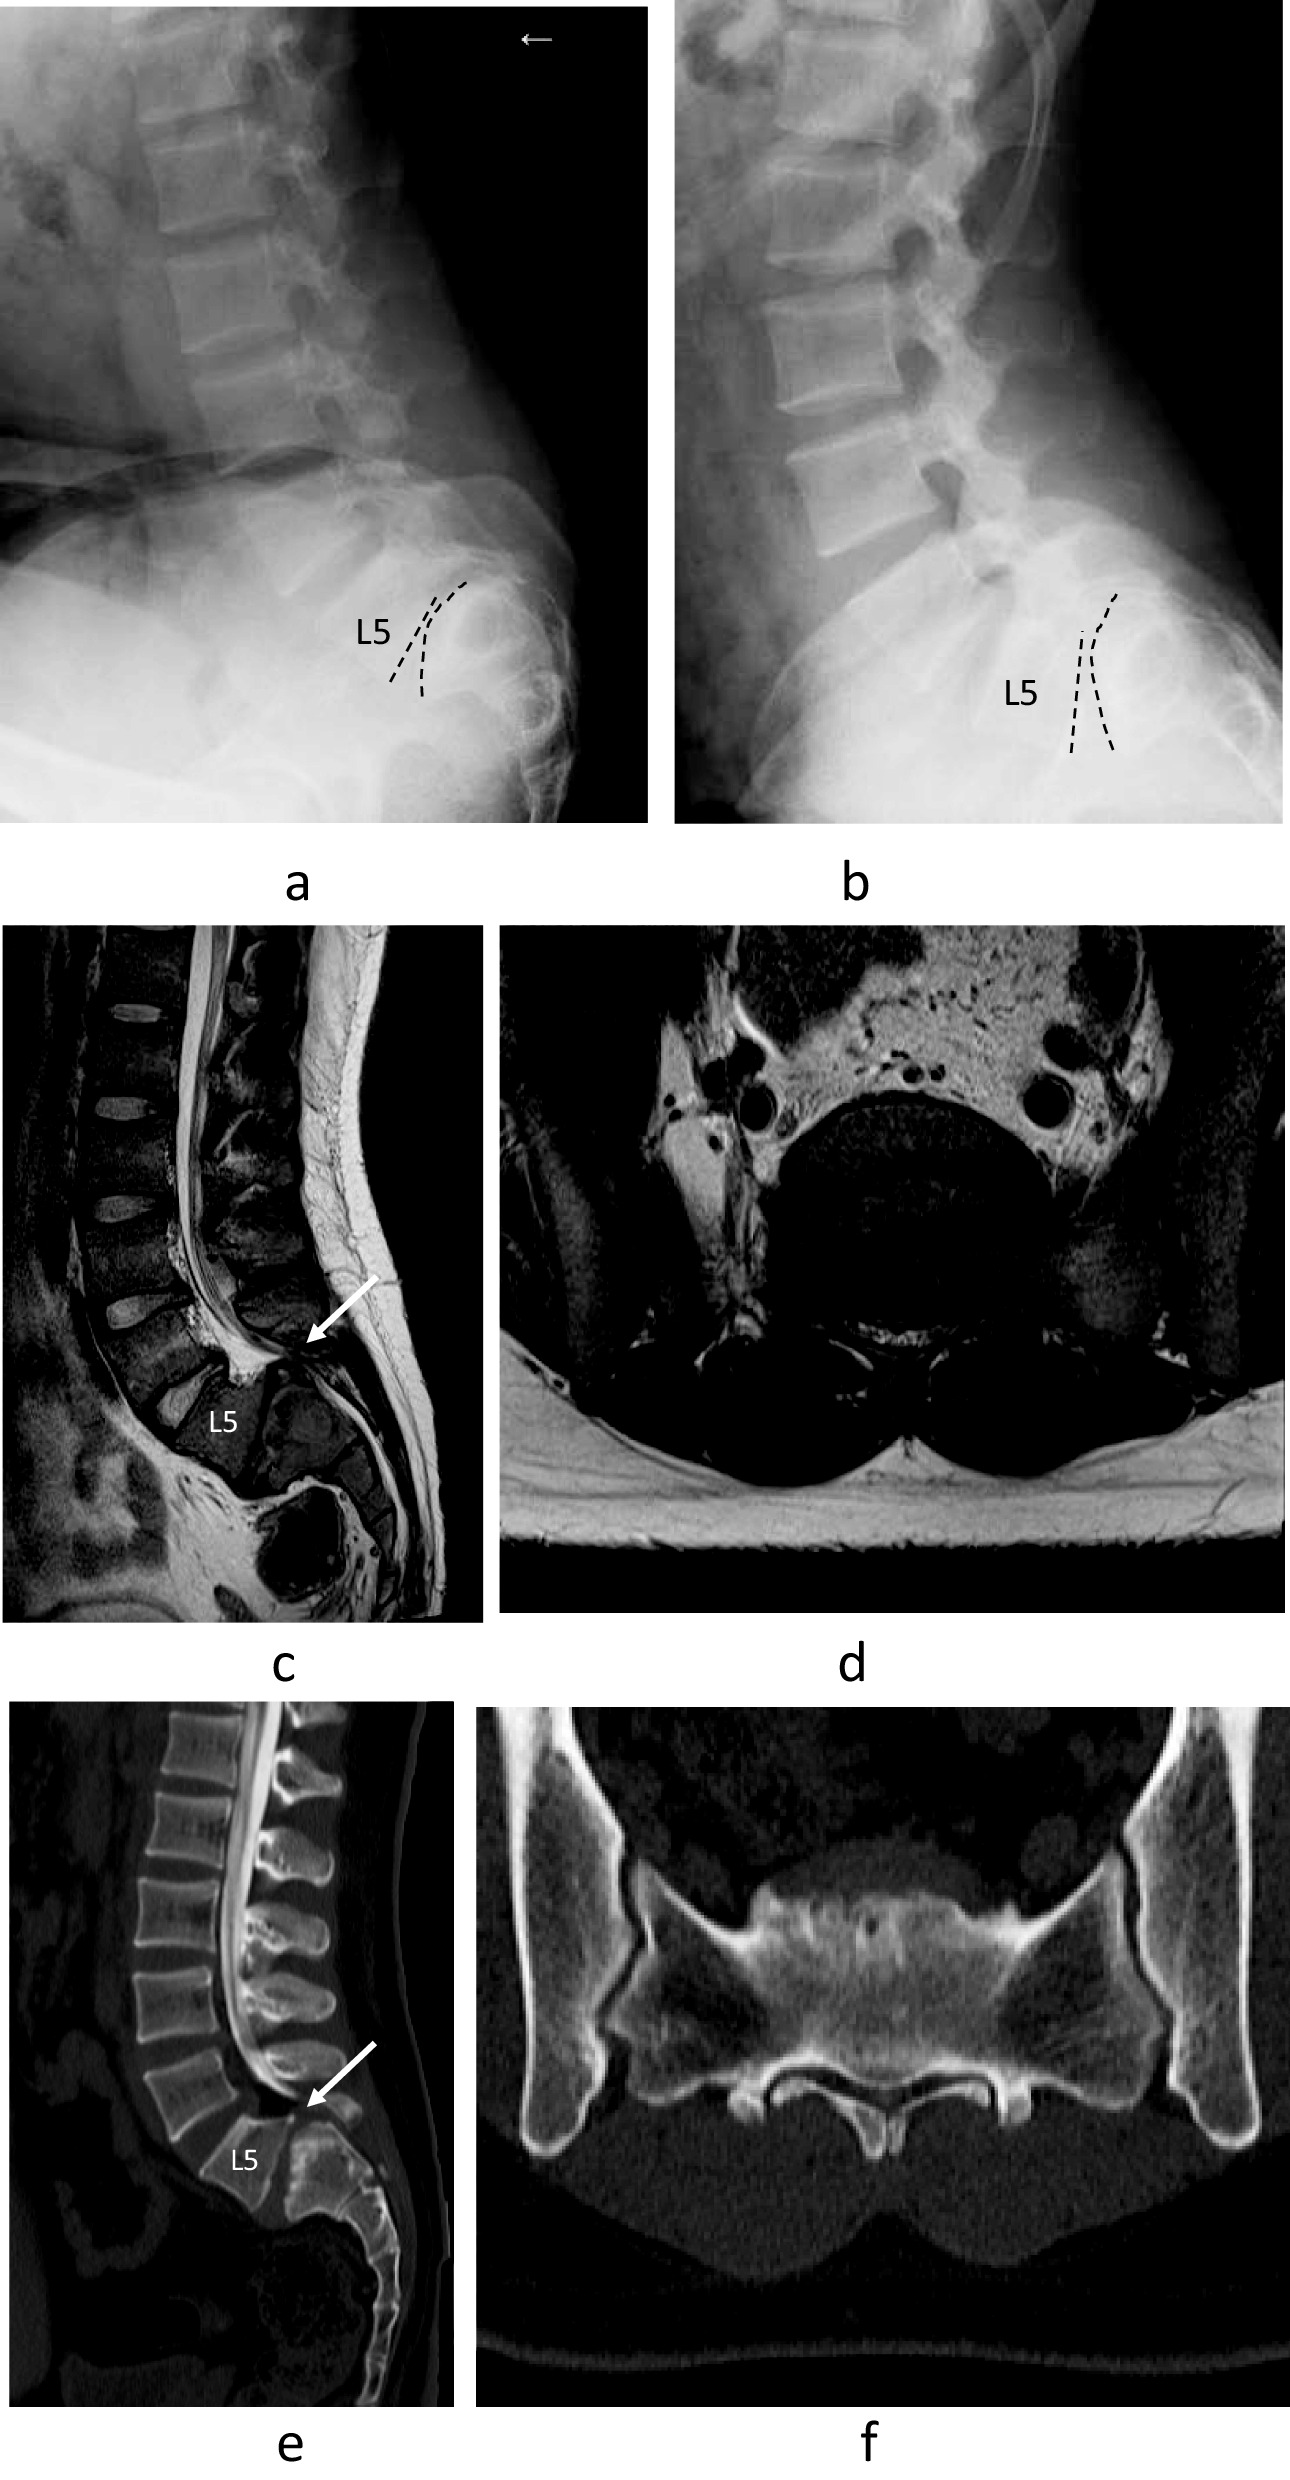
Lateral-view functional plain X-ray in the older brother showing slight instability between L5/S1 flexion and extension (a, b). Magnetic resonance images showing the L5 vertebra slipped anteriorly on S1 and the L5/S1 disc bulging apparent on the sagittal slice (arrow) (c). MRI also showed serious canal stenosis at L5/S1 with disc bulging apparent on the axial slice (d). Computed tomography with myelogram showed a vertically displaced anterior slip to S1, a round-shaped S1 cranial endplate and spinal canal stenosis (arrow) with dysplastic bilateral facets at L5/S1 (e, f)
